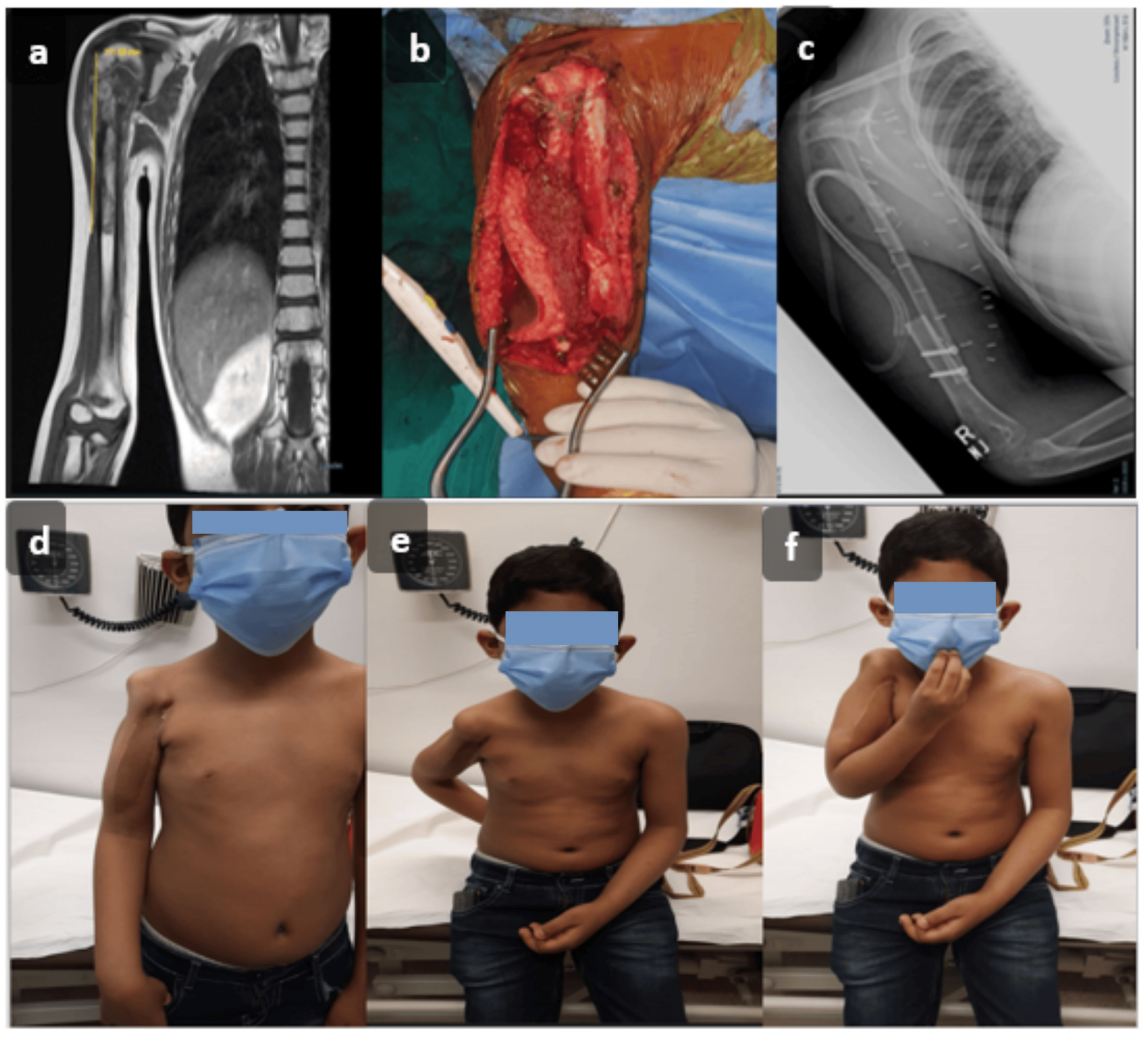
(a) Eight-year-old child with right humerus osteosarcoma seen on magnetic resonance imaging (pre-operative). (b) Right humeral bone defect after placement of free fibula flap with head (blue star). (c) Post-operative plain radiograph with the fibula in place. (d-f) Images showing function of upper limb (Photography and Designed by: Safdar Ali Sheikh).
